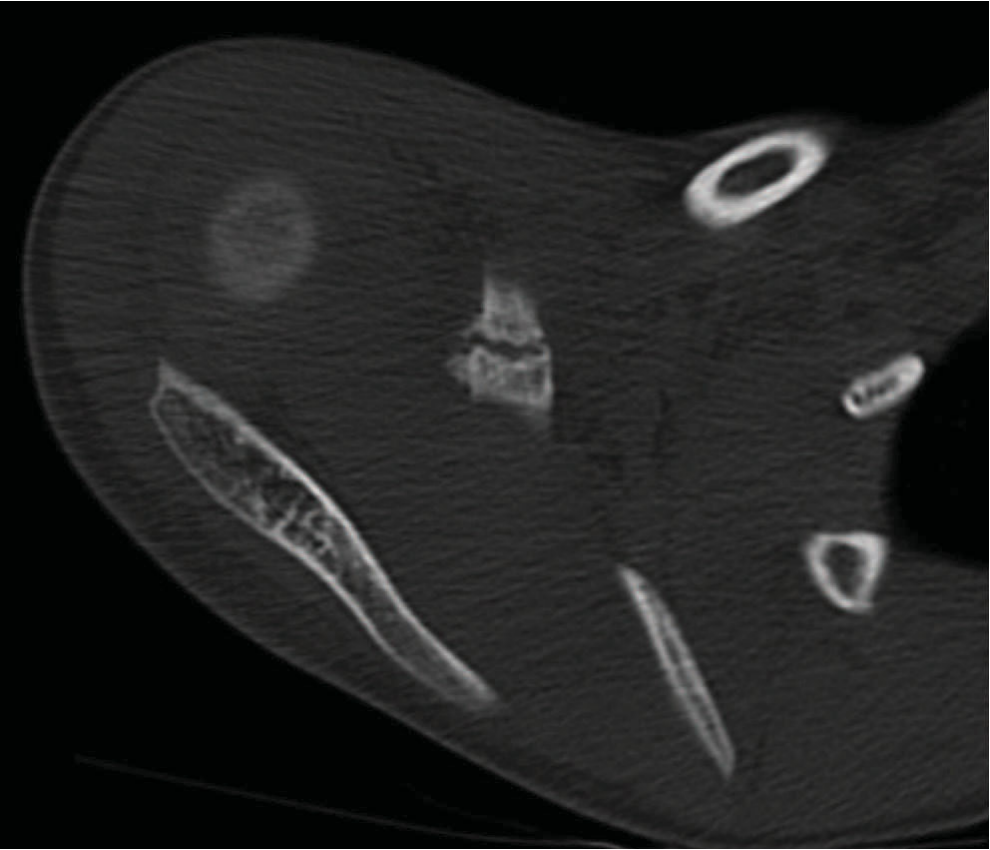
Axial CT image of the right shoulder with an intact epiphyseal plate of the coracoid process.
